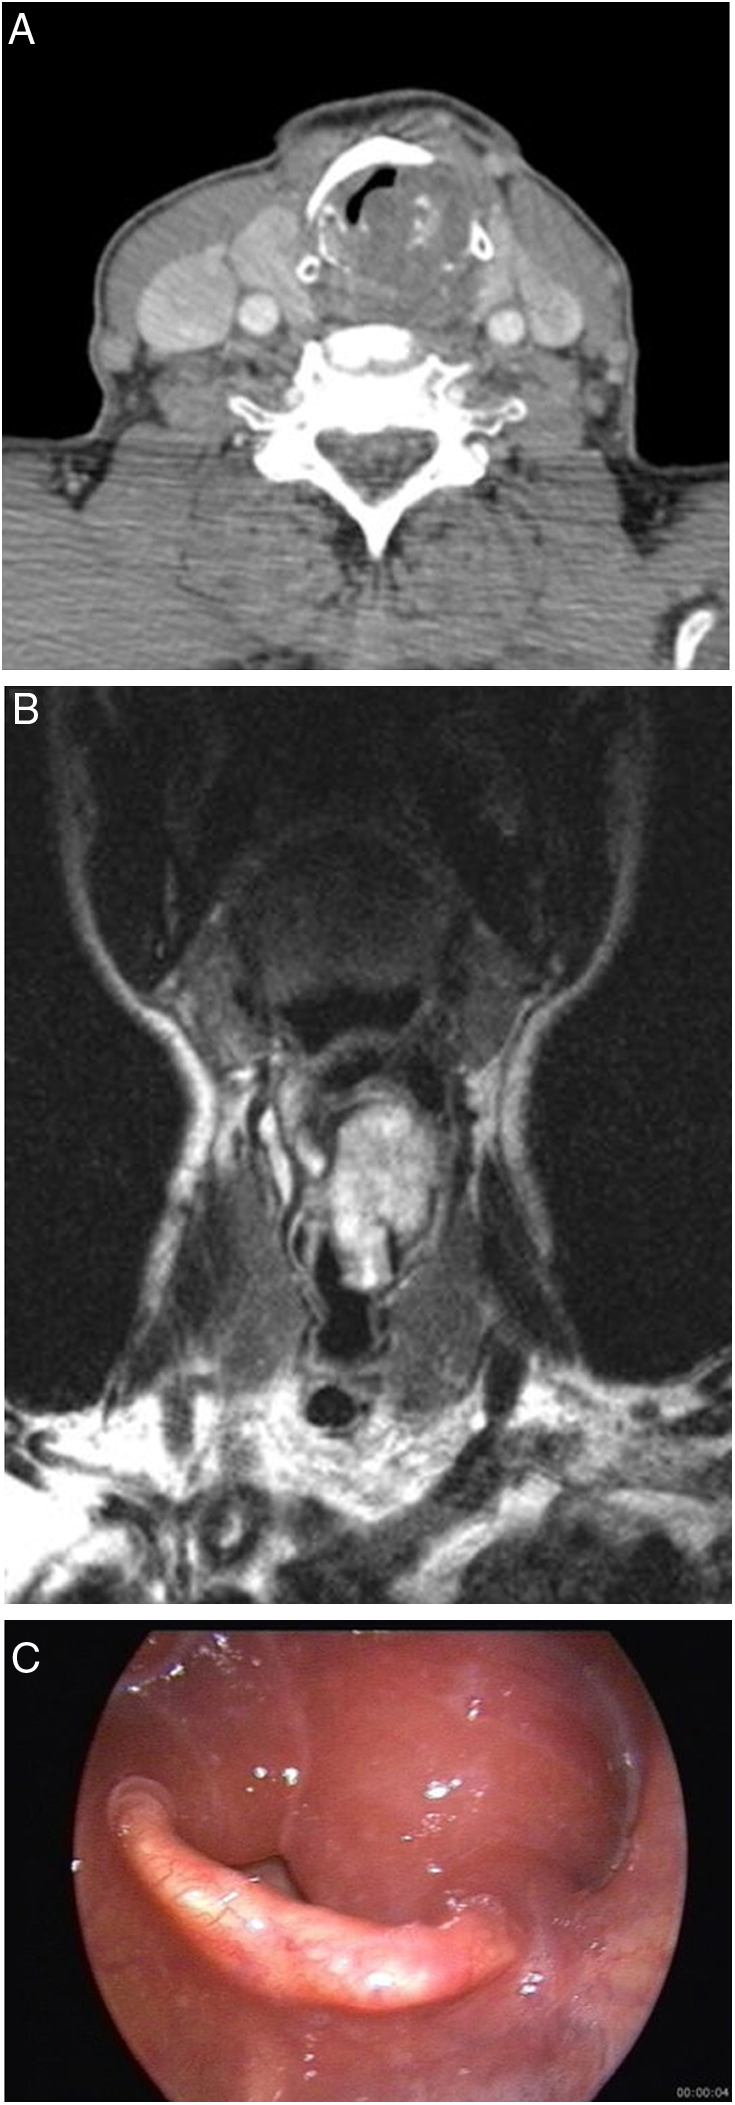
(A–C) 70 year-old man with laryngeal chondroma. Contrast enhanced CT image (A) shows expansile mass arising from cricoid cartilage. Chondroid calcifications are seen within the mass. Coronal T2 weighted TSE image (B) shows high signal intensity transglottic mass. Endoscopy (C) reveals bilateral edema of the mucosa of the arytenoid cartilages and a large submucosal mass.
